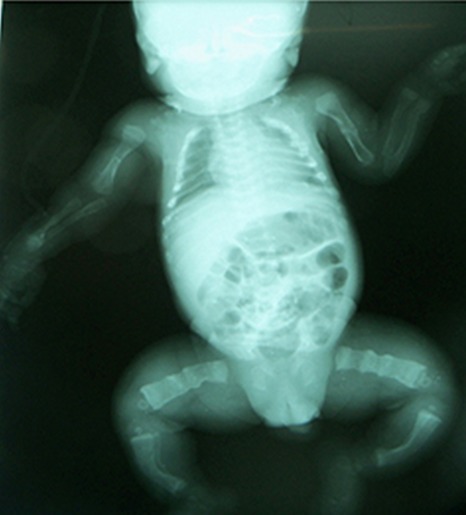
Radiographie thoraco-abdominale et des membres de face: déminéralisation de l'ensemble de la trame osseuse; fractures des os longs des membres avec cals vicieux; fracture du 1/3 supérieur du fémur gauche
